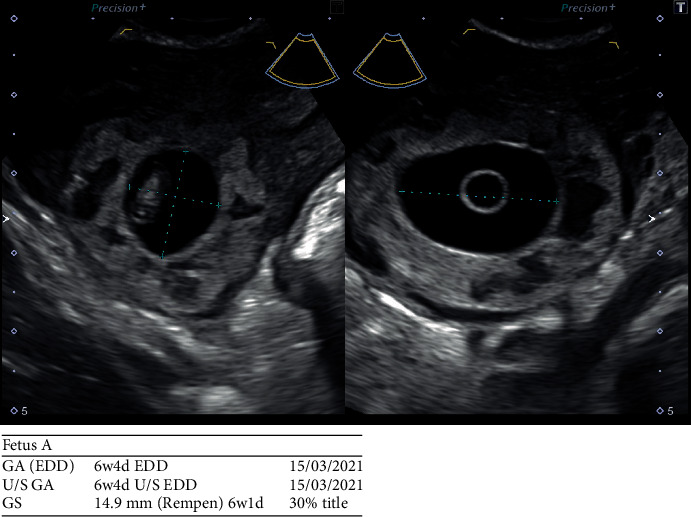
Ultrasound image of the ectopic gestational sac.
